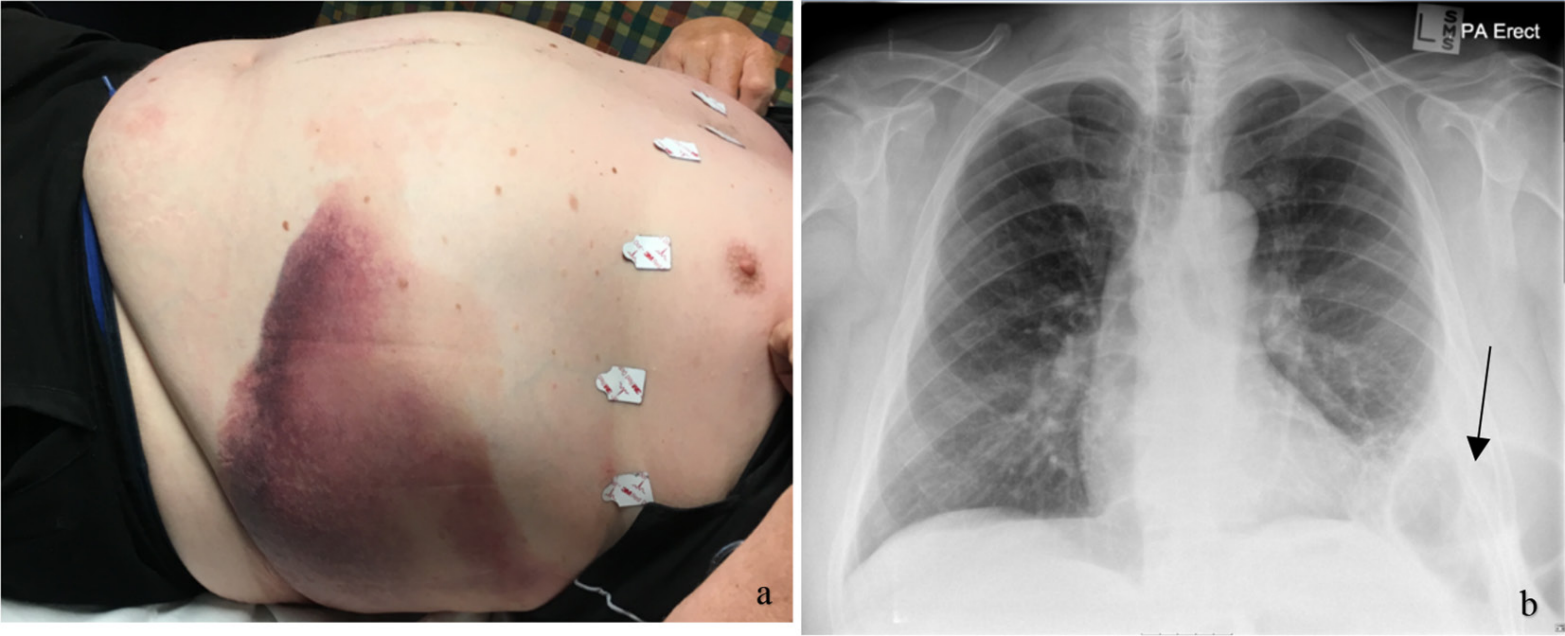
(A) Left upper outer quadrant ecchymosis with bulging of the lower intercostal spaces. (B) A posteroanteriorchest radiography demonstrating a loop of bowel through the intercostal space, suspicious of an intercostal hernia (black arrow).
